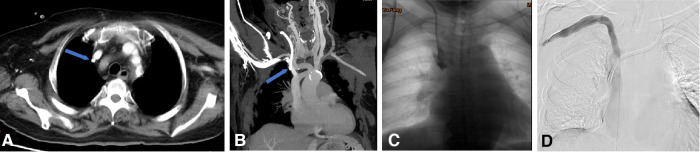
Endoluminal treatment for central venous perforation during dialysis catheter insertion. (A,B) The computed tomography thorax's transverse plane and coronal reformat showed the perforation site outside the right innominate vein (blue arrow). There was no attendant hemo/pneumothorax that otherwise required immediate intervention. (C) The central venogram showed contrast outlining in the mediastinum, confirming the CT findings of catheter perforation of the right innominate vein. (D) A covered stent was subsequently used to cover the rupture when the catheter was pulled into the internal jugular vein. Next, the right subclavian venography showed blood flow smoothly in the stent without contrast extravasation. Then, a new cuff CVC was inserted from the internal jugular vein into the superior vena cava.
